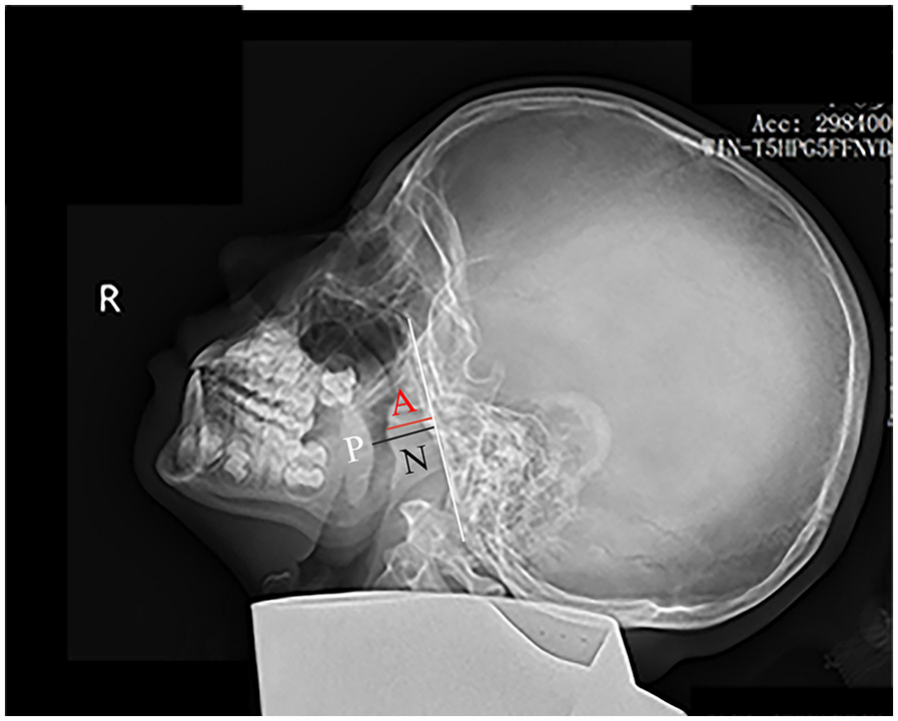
Measurement of the *A*/*N* ratio on a lateral nasopharyngeal x-ray. The white line marks the straight portion of the basiocciput's anterior margin. The adenoid depth (AD) is measured as the perpendicular distance from this line to the point of maximal adenoid prominence (red line). The nasopharyngeal depth (ND) extends from the posterior hard palate (P) to the anteroinferior edge of the sphenobasioccipital synchondrosis (black line).
